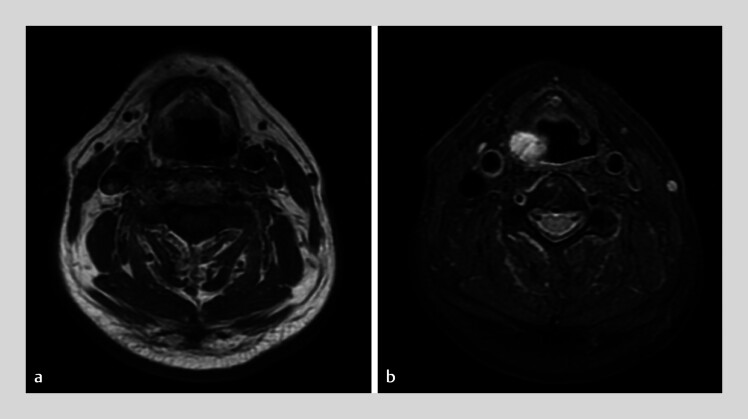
Appearance of the lesion on magnetic resonance imaging.
